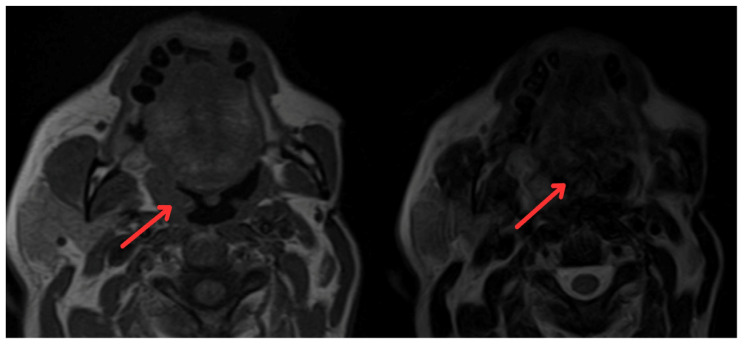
Magnetic resonance imaging of paranasal sinuses showing irregular enhancement forming a mass that involves the mucopharyngeal space and the right palatine tonsil (red arrows).
